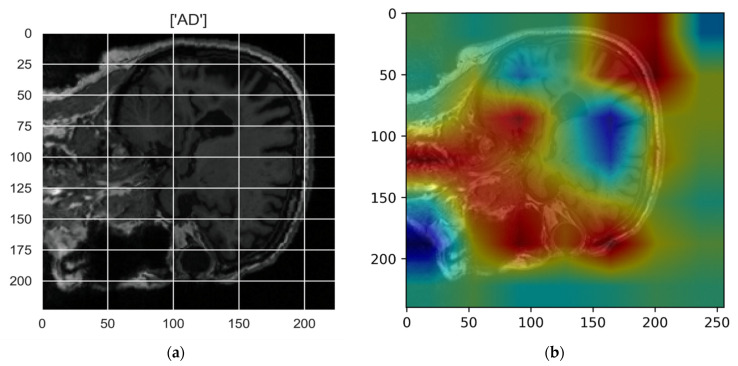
Grad-CAM explanation for the prediction of class AD: (a) MRI image, (b) Grad-CAM attention map.
